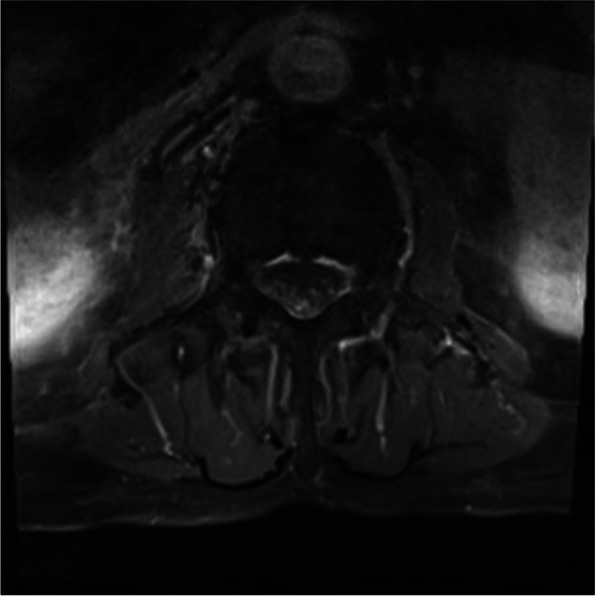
T1-weighted gadolinium-enhanced axial MRI at L4–5 level performed 3 weeks after the onset of symptoms due to cytomegalovirus polyradiculitis showing the enhancement in the entire lumbosacral nerve root
